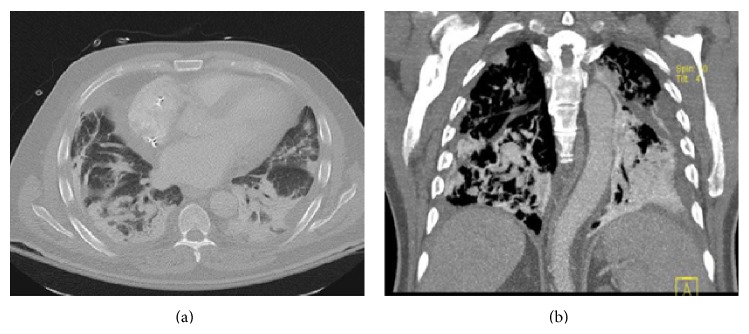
(a) and (b) CT chest with contrast showing high attenuation areas of multiple conglomerate consolidation in both lung bases ((a) axial cut; (b) coronal cut).
